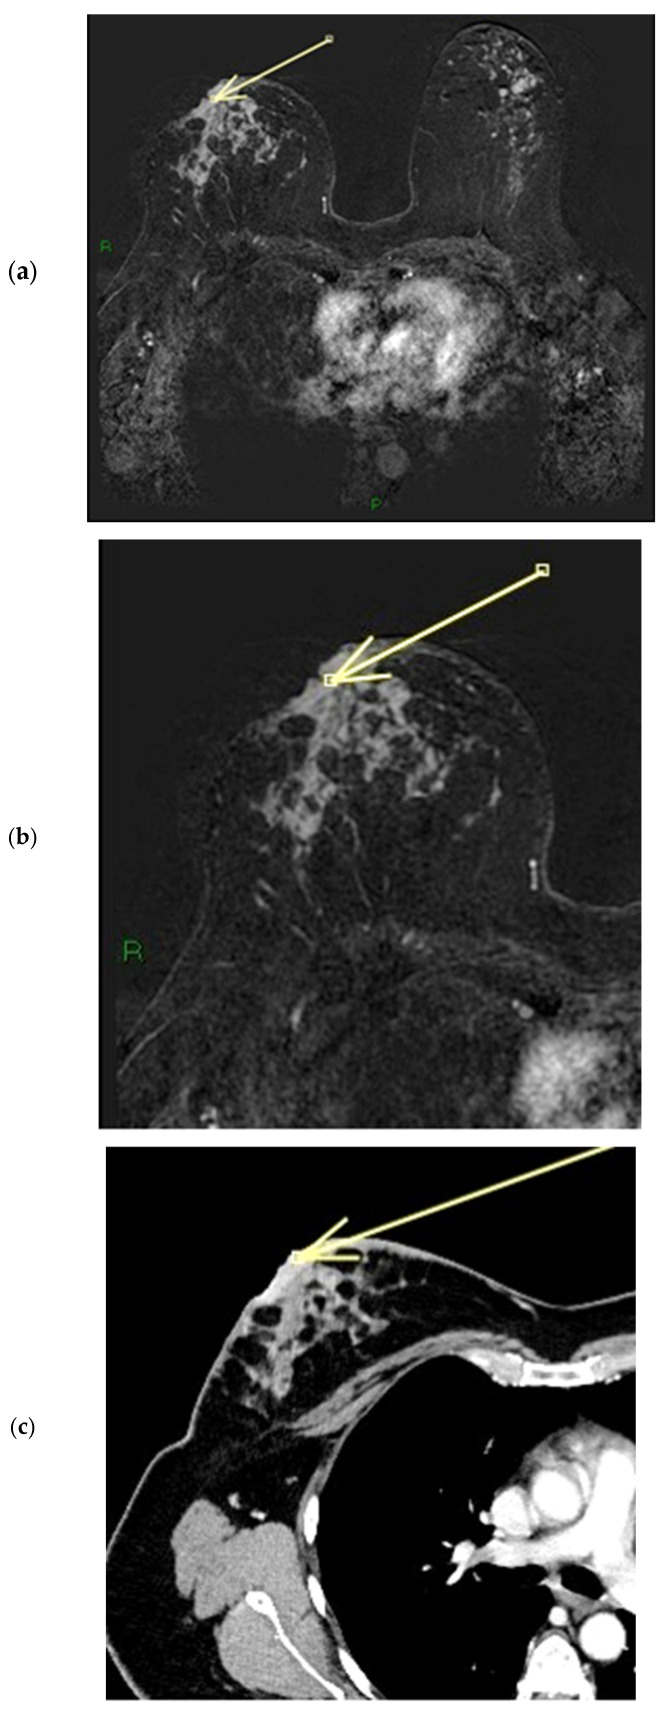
(a–c) Patient’s name DN.A. The external quadrants of the right breast are occupied by a mass lesion with non-mass peripheral components, which infiltrates the nipple, retracting it. During ce-MR, we see a flat thickened right nipple, with SLE, NEZ, and marked patchy INE pattern of enhancement (arrows in a,b). In the ce-CT scan, the arrow shows enhancement of both the base and the body of the right nipple. Morphology is inverted, with skin thickening and ulceration (c).
